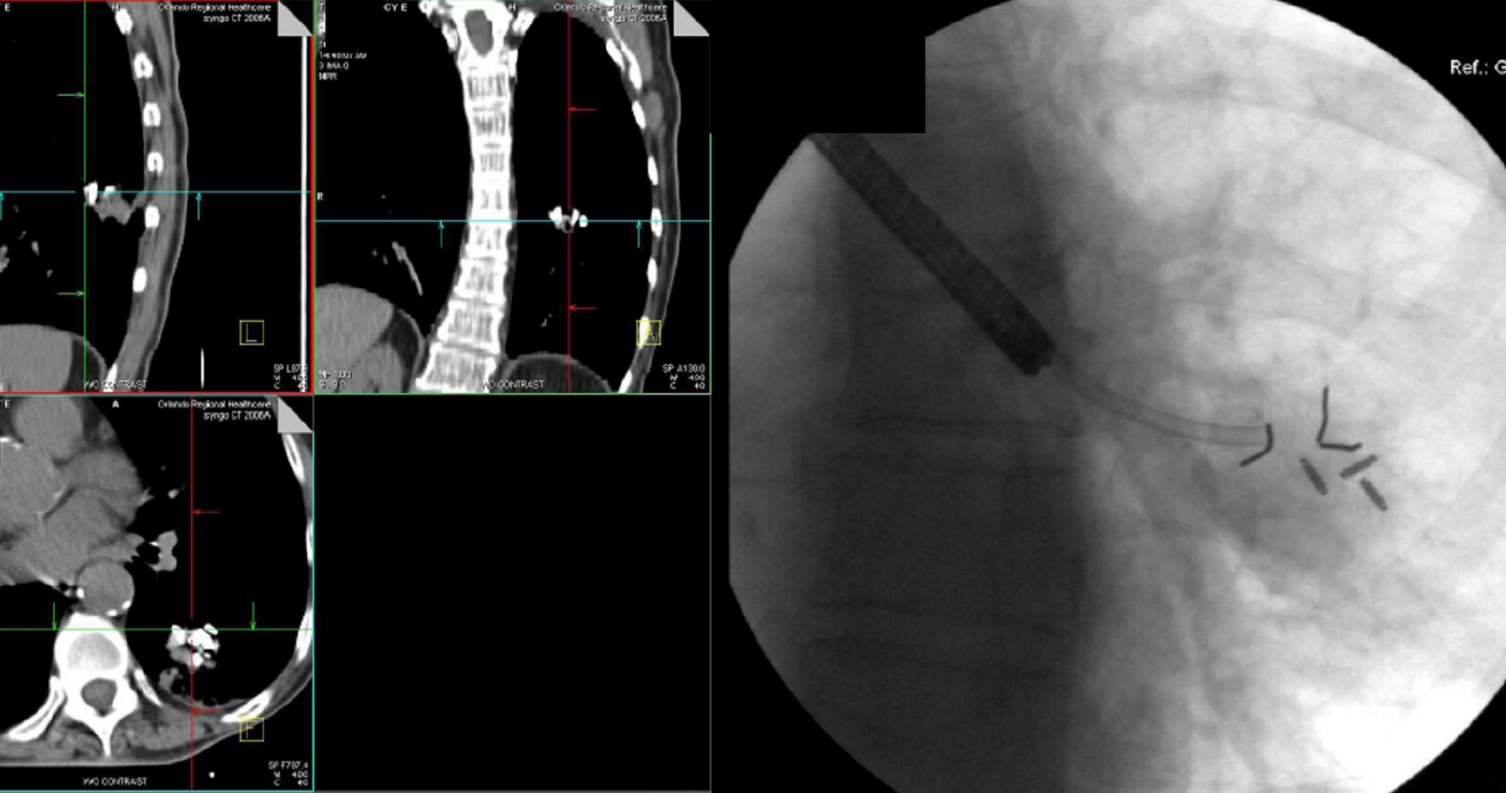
Images from lung cancer patient with left lobe, posterior lesion approximately 1.5 cm in diameter with three RF transmitters and two gold fiducial markers implanted. The fiducial markers were placed to ensure device stability. RF transmitter devices and fiducial markers imaged on (a) computed tomography and (b) a c‐arm fluoroscopic system.
